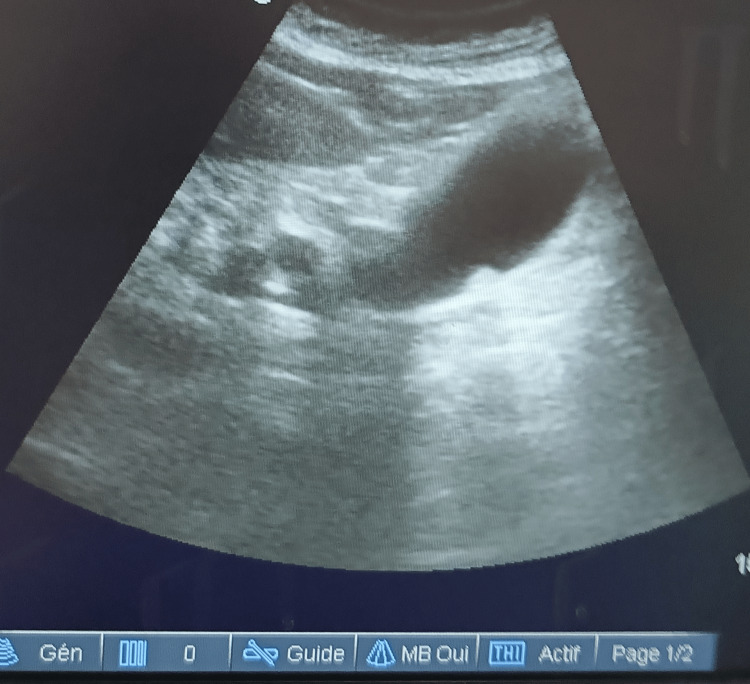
Abdominal POCUS showing multiple gallbladder calculi with sludge but without visible biliary distention POCUS: point-of-care ultrasound
